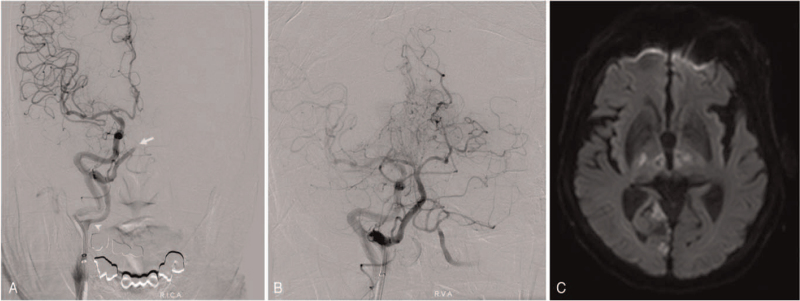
(A) Distal subtraction angiography shows the distal basilar artery occlusion (white arrow). The origin of the persistent primitive hypoglossal artery is at the proximal cervical segment of the internal carotid artery (white arrowhead). (B) Postprocedural angiography shows complete recanalization of the occluded basilar artery. (C) Postprocedural magnetic resonance imaging of the brain reveals multifocal cerebral infarctions in the bilateral thalamus, midbrain, and cerebellar vermis.
